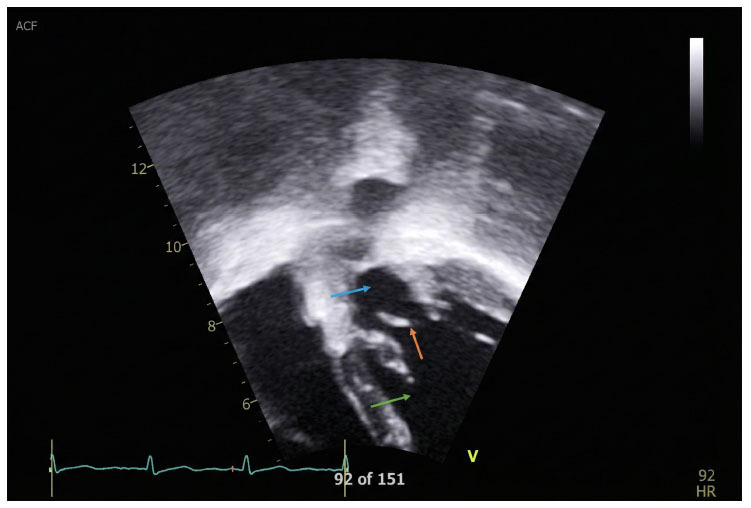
A transesophageal 2D echo with apical 4-chamber view showing the false tendon (orange arrow) during diastole traversing the mitral valve from left atrium (blue arrow) to left ventricle (green arrow).
